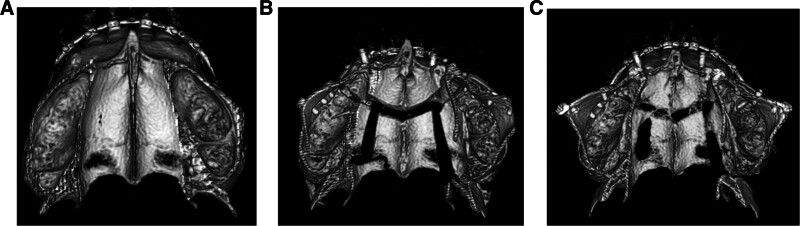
CT reconstruction at the level of palate. (A) Presurgery, (B) immediately postsurgery, (C) 3 months postsurgery.
